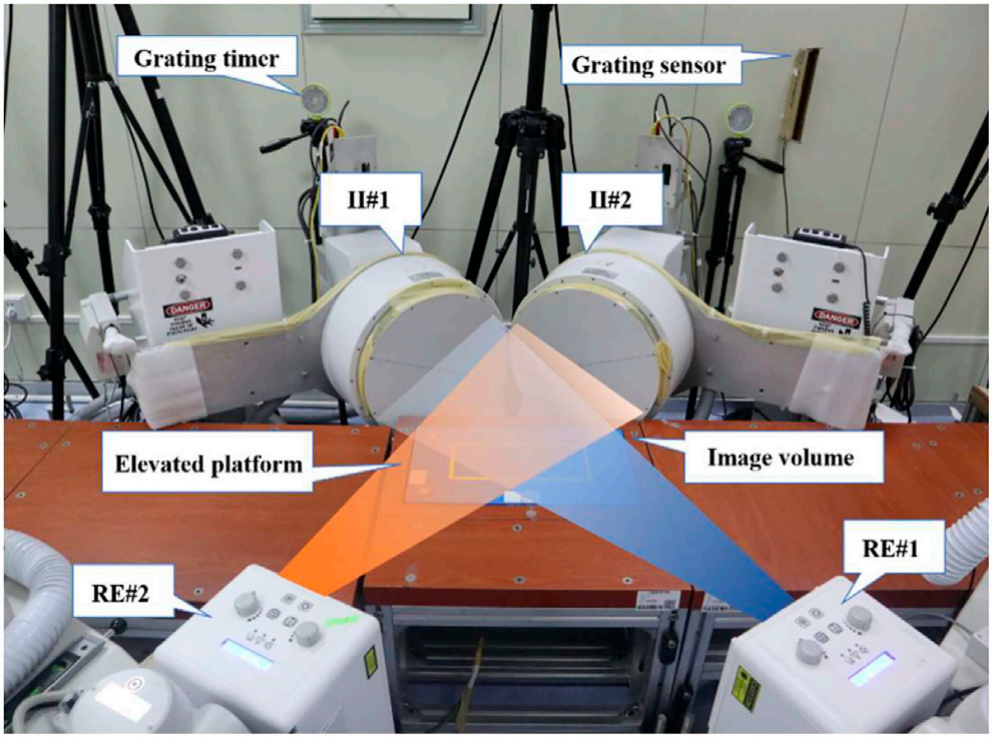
High-speed DFIS set-up. Participants ran on an elevated platform. Image intensifiers (II#1 and II#2) processed images created by X-rays from the radiographic emitters (RE#1 and RE#2).
